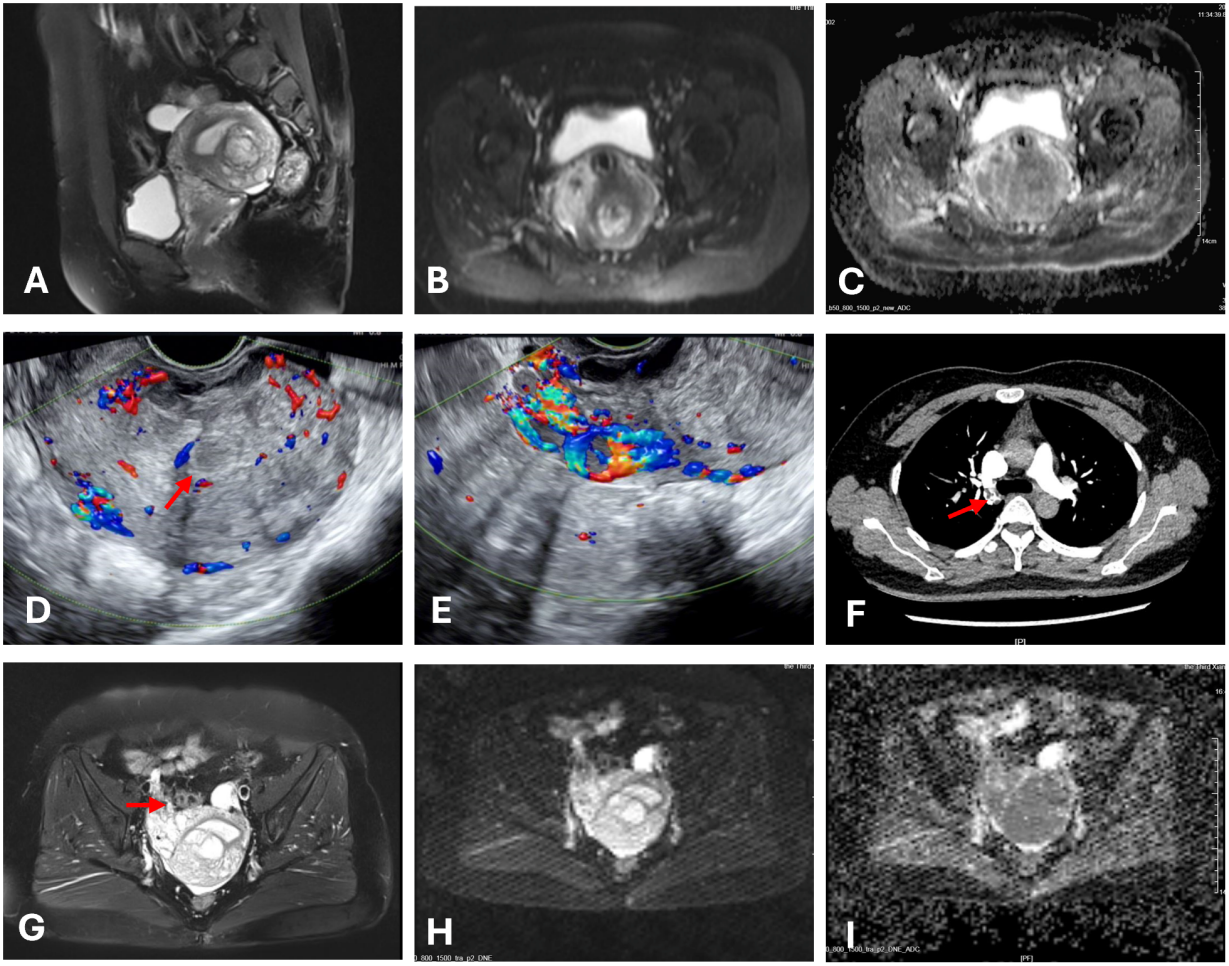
Images of the mass. The red arrow indicates the mass. (A) Multiple nodular lesions in the posterior uterine wall. (B, C, H, I) The tumor showed high signal on DWI and low signal on the ADC. (D) Ultrasonography shows multiple ill-defined hypoechoic nodules coalescing in the myometrium. (E) Diffuse linear hypoechoic nodules along arcuate vessels (anterior uterine wall) and right parametrial vessels, with increased blood flow signals. (F) CTA showed filling defects within the right pulmonary artery. (G) Nodular and tortuous tubular structures which shares similar MRI signals with the uterine mass were suggestive of angioleiomyoma.
